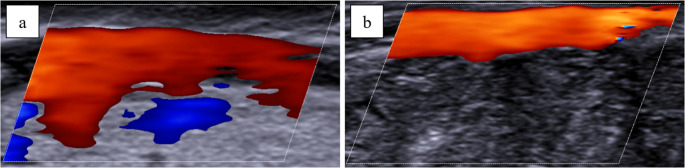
Longitudinal scan of the anterior interventricular artery (a) and of the right coronary artery (b). Color Doppler ultrasound revealed steady blood flow within both vessels
